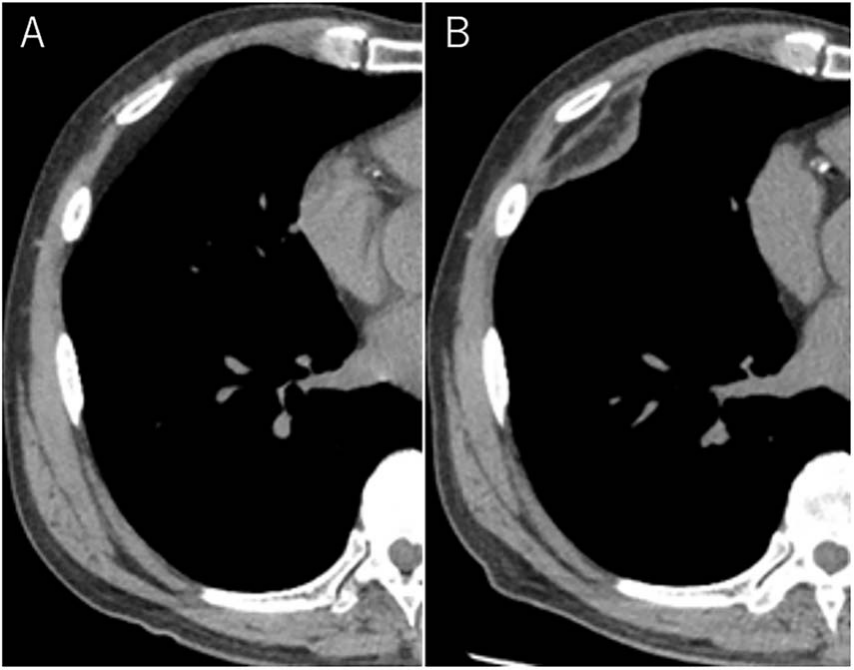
Mixed density mass located in front of thoracic wall; the size was 8.0 × 5.0 × 3.0 cm (B); (A) The tumor seen as thoracic wall fat (7.8 × 4.8 × 1.2 cm) (arrow) on CT 1 year ago; (B) the size increased and the density has changed to heterogenous in 1 year.
